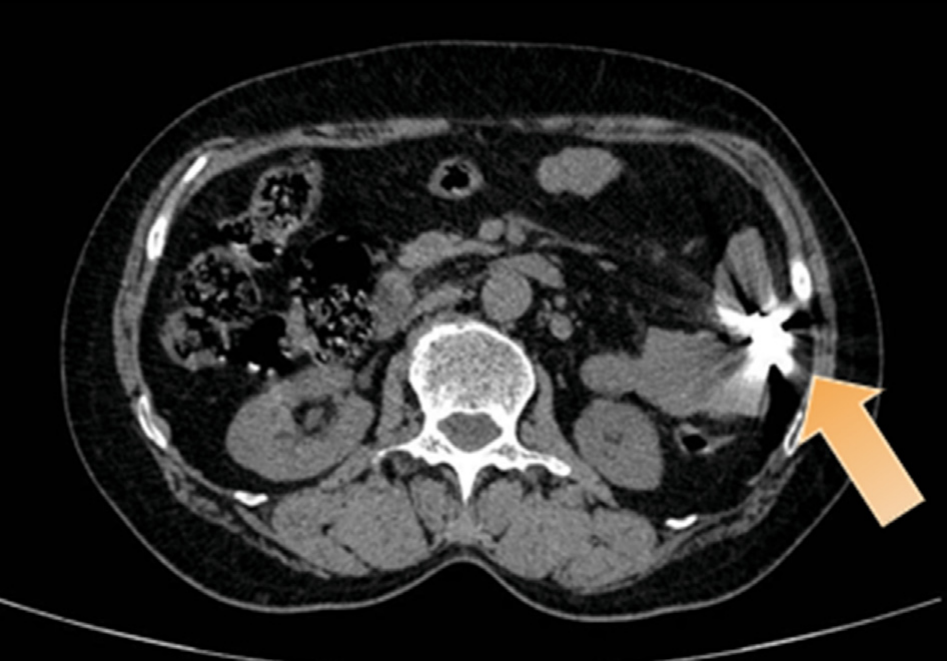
Abdominal CT identified the dental prosthesis in the jejunum.
